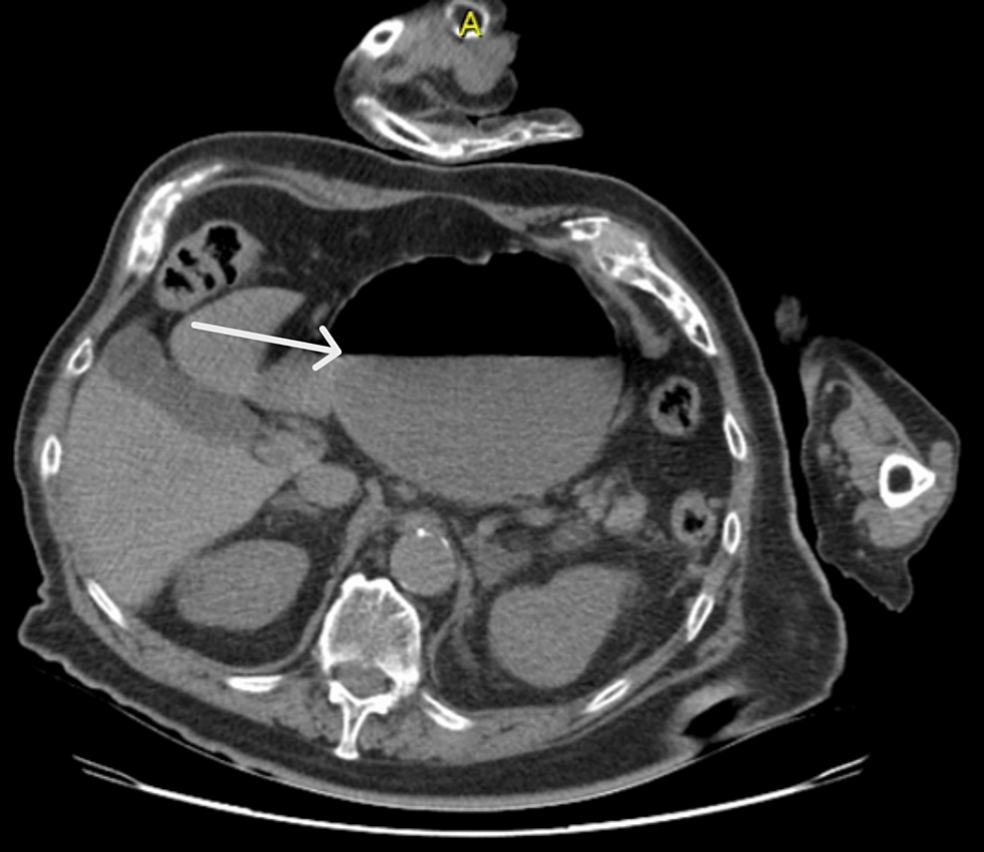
CT scan of the abdomen in the axial plane, showing a distended stomach with a double-pouch appearance and air-fluid levels (white arrow)
